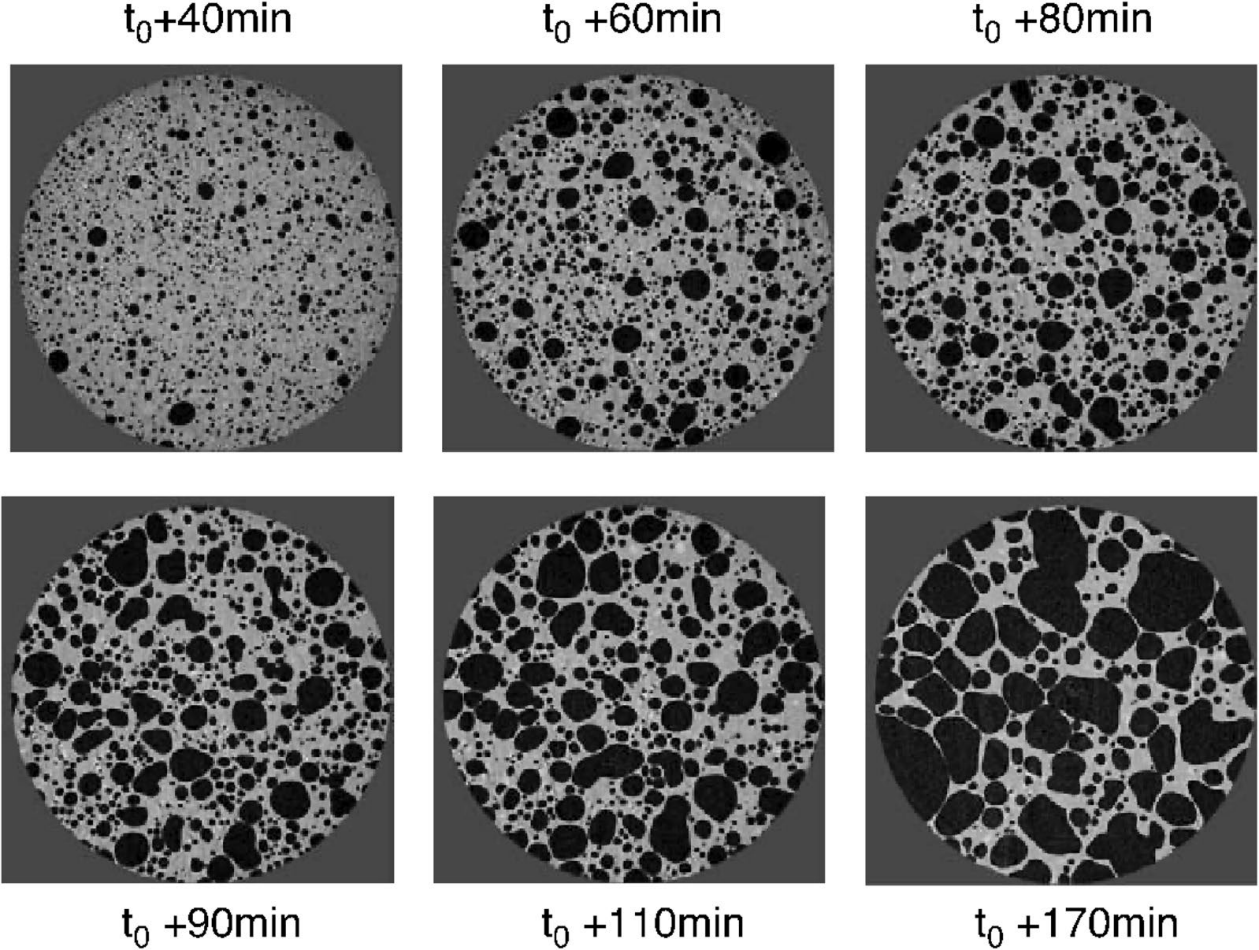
Dynamic bubble growth at increasing time period in dough in reconstructed synchrotron 3D X-ray images slices [44]
